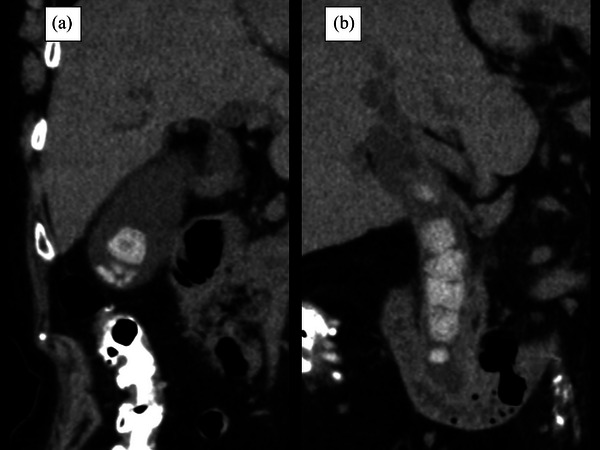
Abdominal computed tomography showing (a) a gallstone measuring 18 mm in diameter and (b) multiple common bile duct stones measuring up to 20 mm in diameter.
